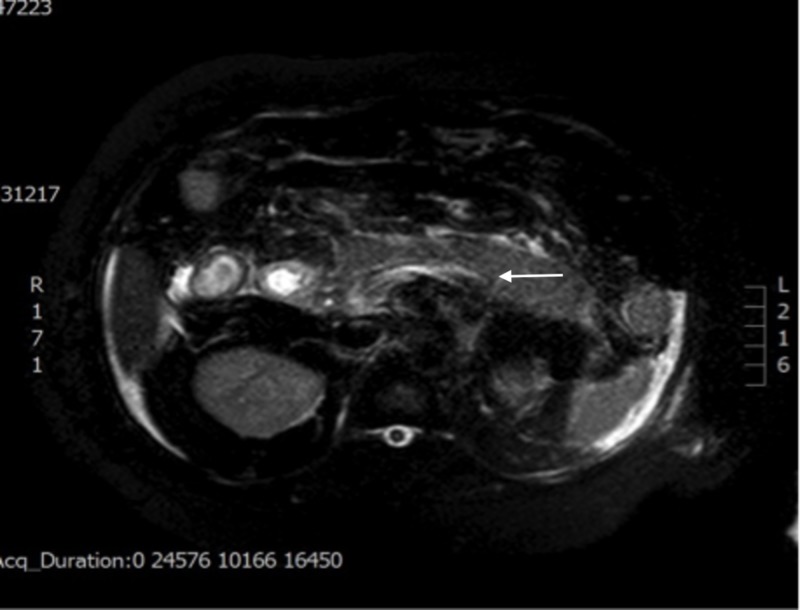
MRCP, axial view, T2-weighted image Peripancreatic stranding consistent with acute pancreatitis (white arrow), normal caliber CBD without intrahepatic or extrahepatic biliary ductal dilatation or choledocholithiasis. MRCP: magnetic retrograde cholangio-pancreatography; CBD: common bile duct.
